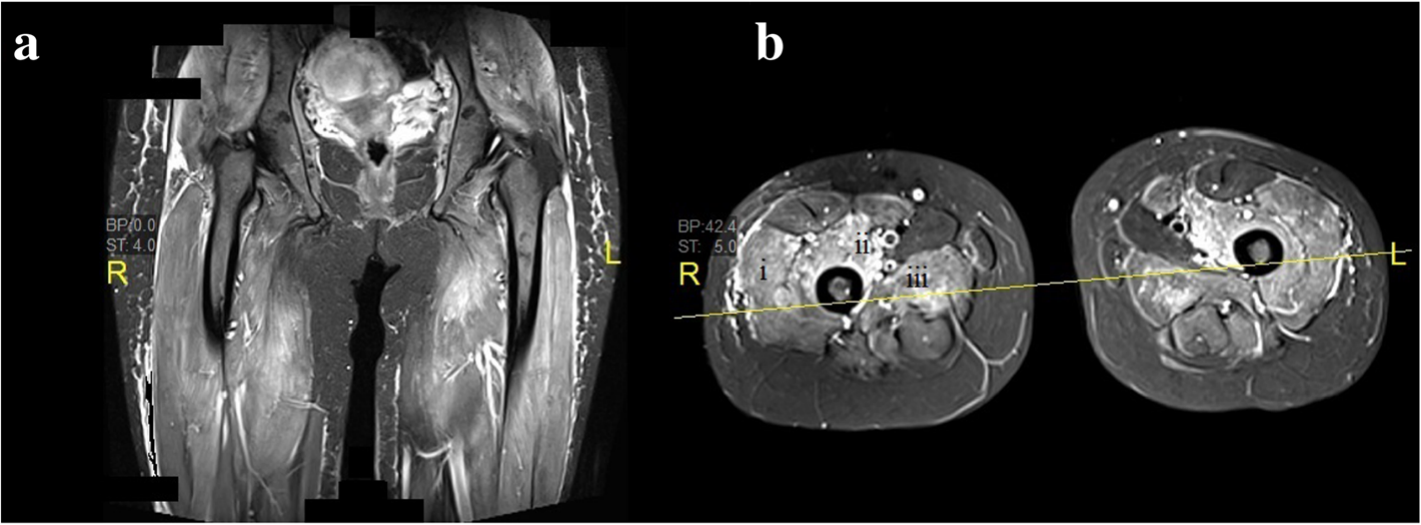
Magnetic resonance images of both thighs. a Coronal short T1 inversion recovery image. b Axial short T1 inversion recovery image. Diffuse hyperintensity in thigh muscles suggestive of edema – (i) vastus lateralis, (ii) vastus medialis, and (iii) adductor magnus
